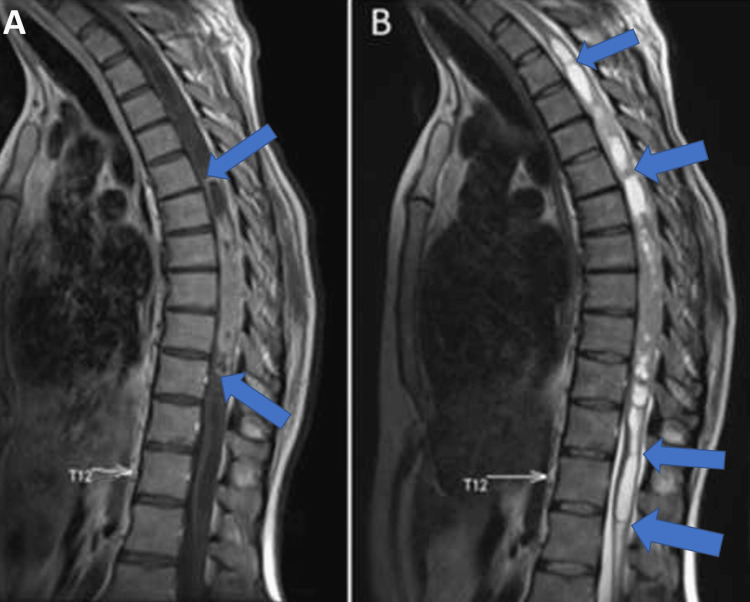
Preoperative MRI of the thoracic spine showing the lesion and the syrinx cavity (A) T1-weighted sagittal scan with gadolinium enhancement showing intramedullary homogeneously enhanced mass extending from the vertebral level of T5 to T10. Blue arrows indicate the borders of the lesion. The white arrow indicates the vertebral level of T12. (B) T2-weighted sagittal scan showing the syrinx cavity (blue arrows). The white arrow indicates the vertebral level of T12.
